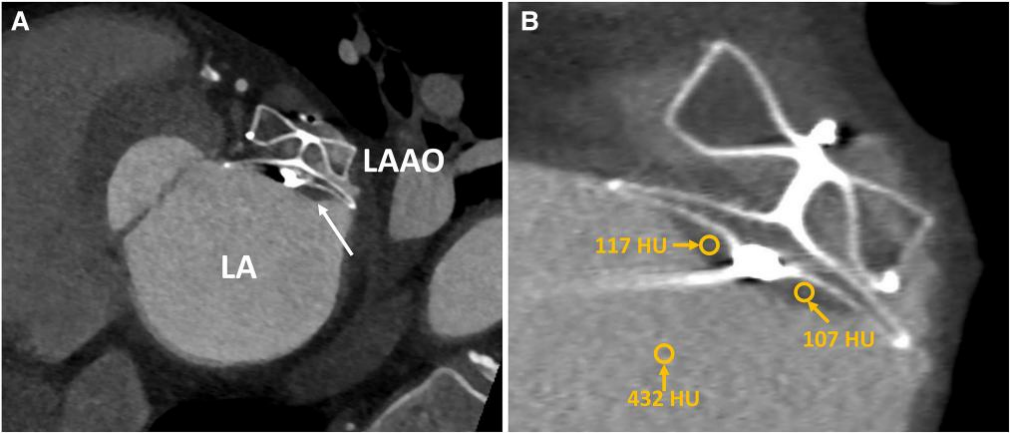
Cardiac computed tomography angiography after left atrial appendage occlusion (LAAO) procedure. *(A*) Hypoattenuated thickening on the atrial device surface consistent with device-related thrombus (DRT) on the device disc. *(B*) Hounsfield unit (HU) measurements of DRT and blood in LA. LA, left atrium; LAAO, left atrial appendage occlusion.
